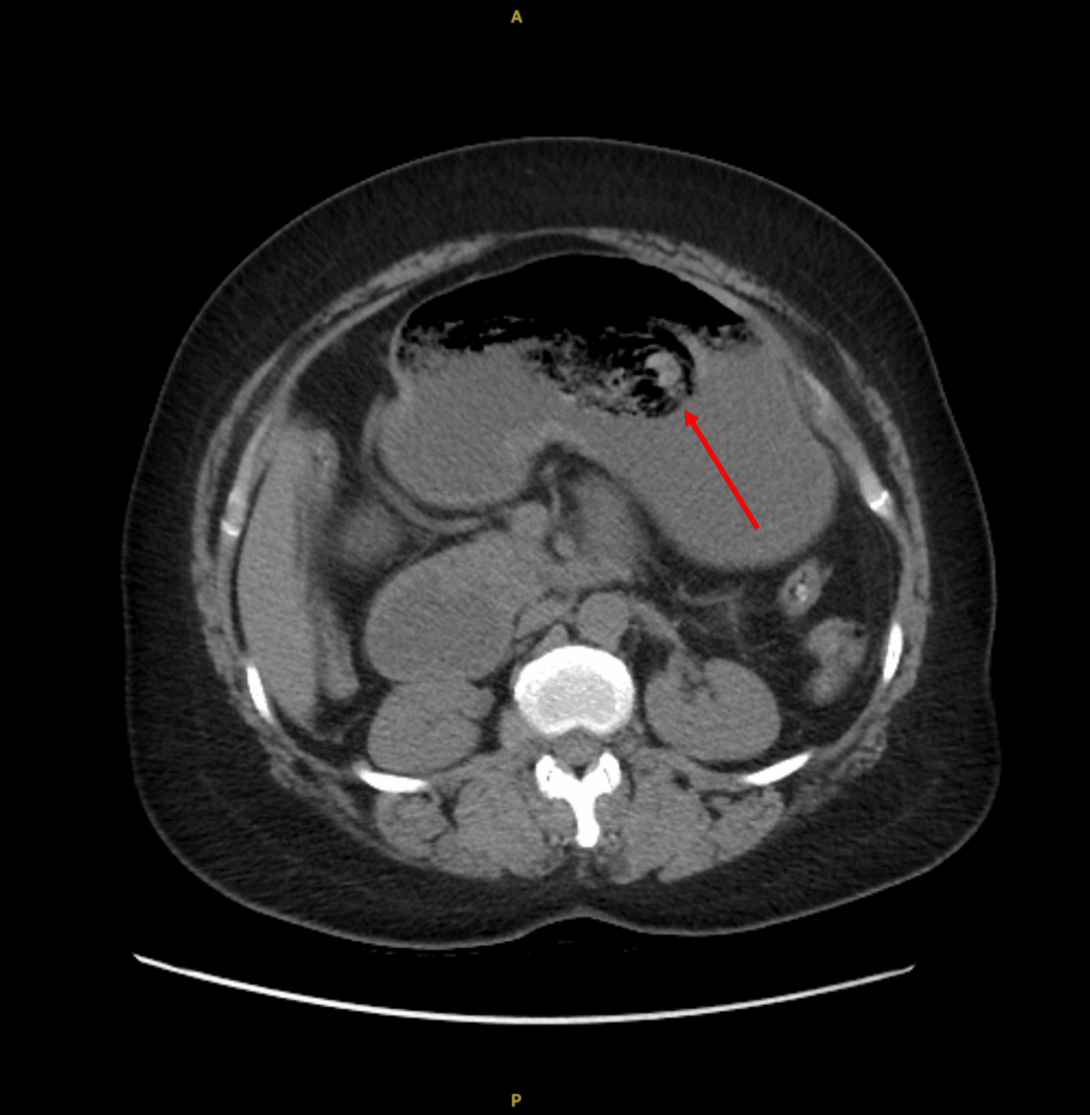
Non-contrast CT scan, axial view, showcasing a large bezoar mass in the gastric antrum (red arrow).
